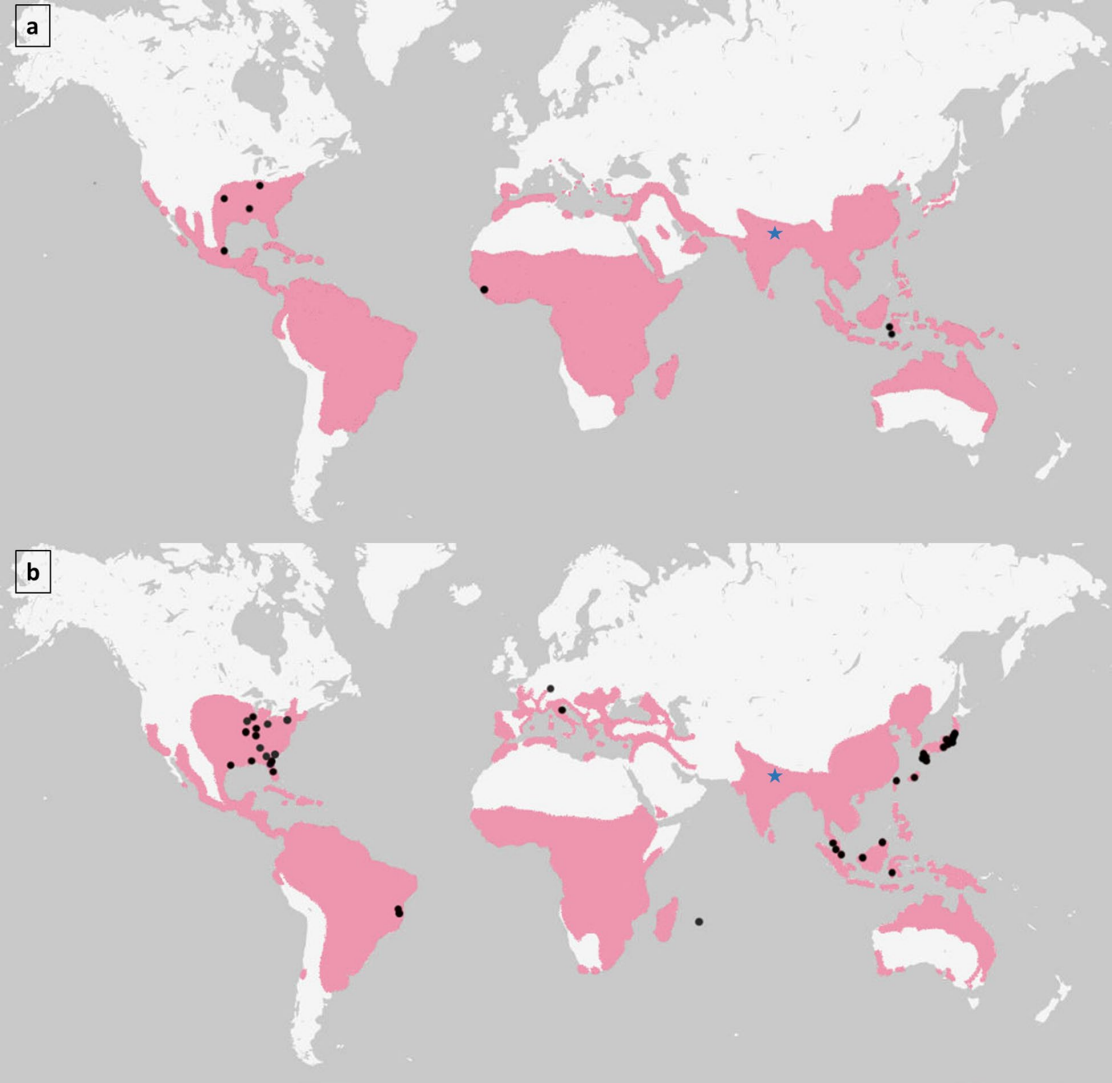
Origin of *Ae. aegypti* (a) and *Ae. albopictus* (b) populations with known cold tolerance (CT). The predicted distribution of the two species according to [47] is shaded in magenta. The origin of populations examined in CT studies included in the meta-analysis are marked with black dots. The sampling site of mosquitoes used for CT experiments in the present study (Chitwan, Nepal) is marked with a blue star (source of map: https://mapstyle.withgoogle.com/)
